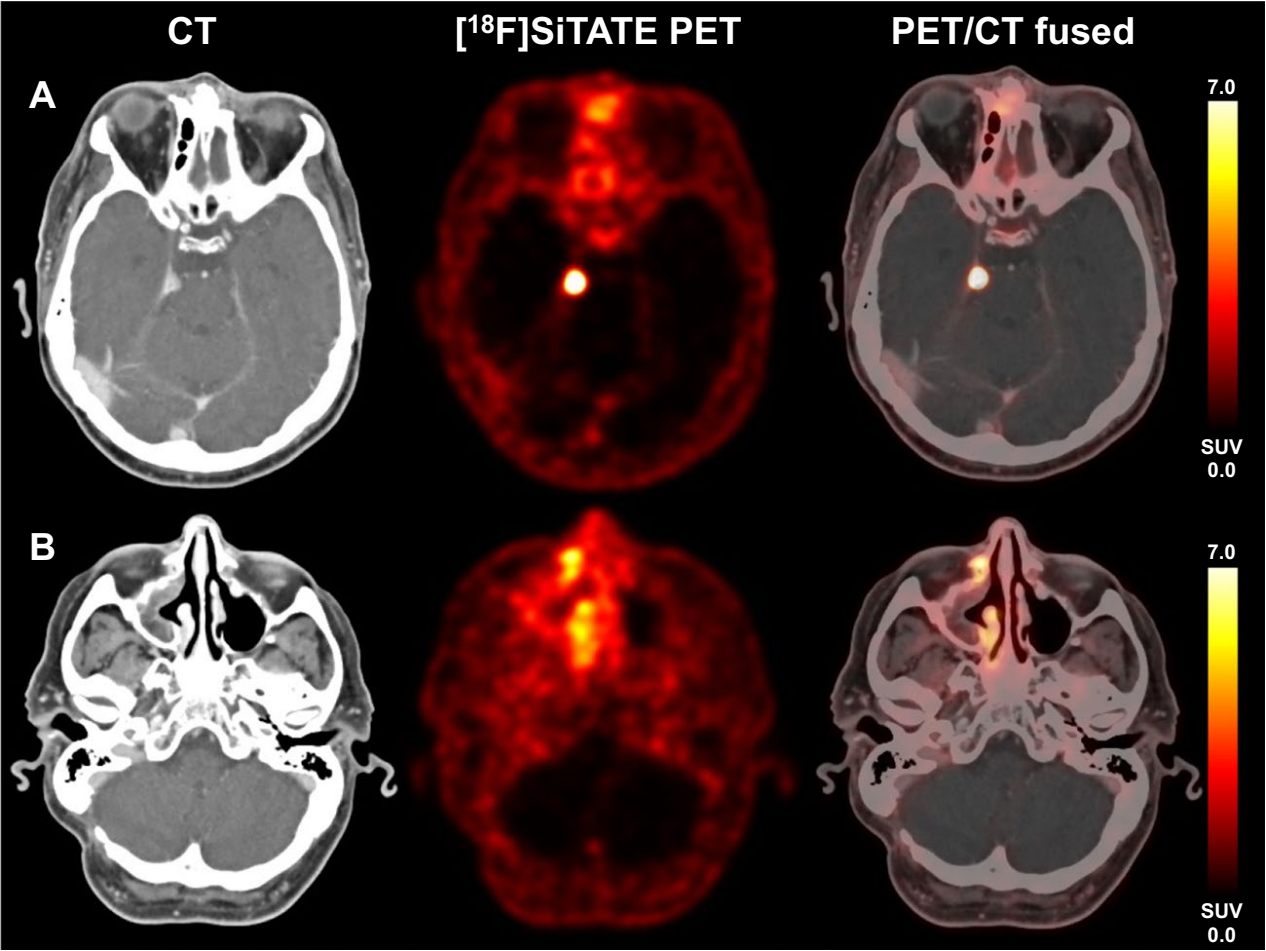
A 64-year-old with residual transitional meningioma, CNS WHO grade 1 at the right tentorium (A) with strong SSTR-expression (SUV_max_ 21.4), but also signs of chronic sinusitis at the right sinus maxillaris (B) with moderate SSTR-expression due to chronic inflammation (SUV_max_ 8.1)
